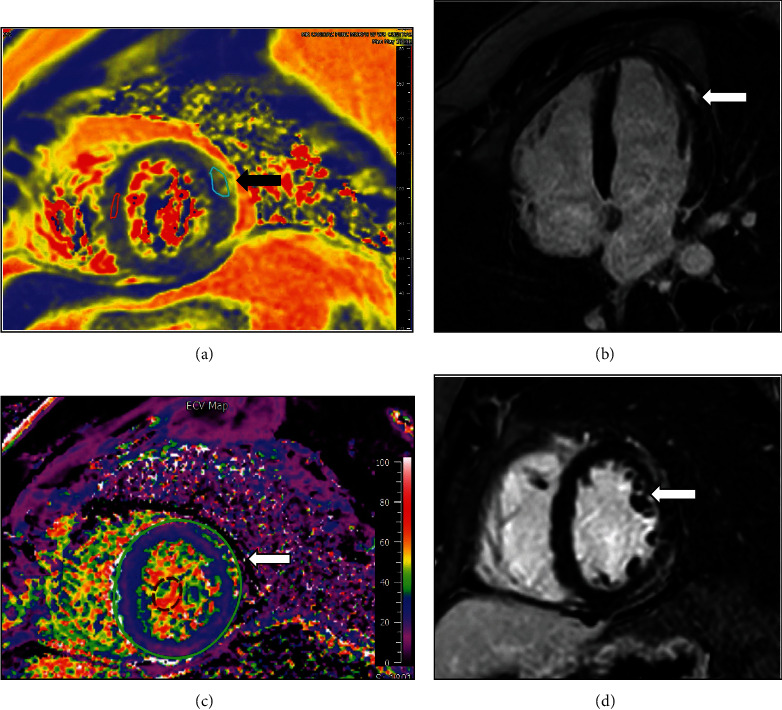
Cardiac MRI of patient C: (a) T2 map demonstrating increased T2 signal consistent with edema in epicardium of the apical lateral wall; (b) four-chamber view showing late gadolinium enhancement in the apical lateral wall; (c) ECV map showing increased signal consistent with edema in the midanterolateral segments; (d) short axis with late gadolinium enhancements in the midanterolateral segments.
